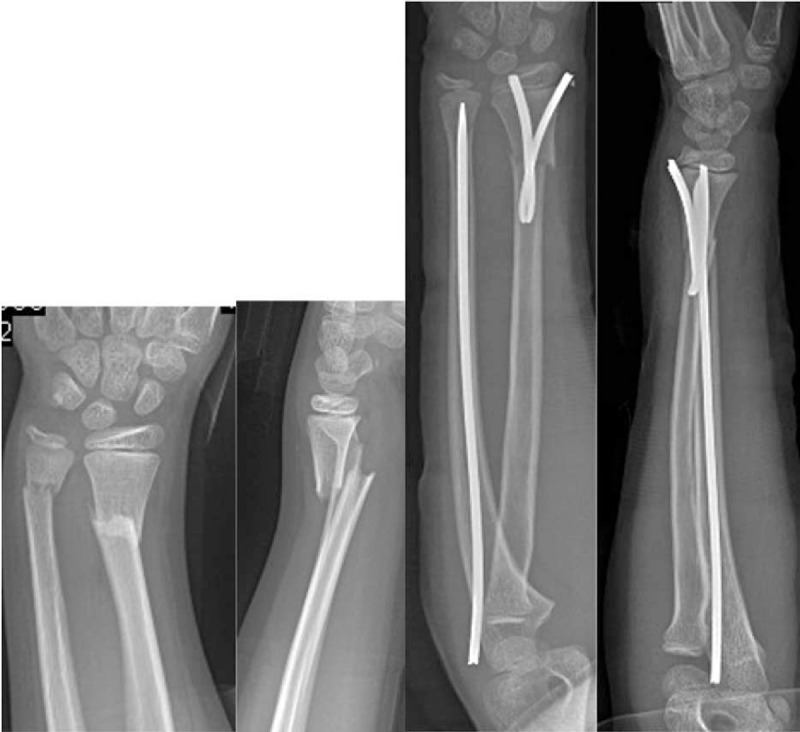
Pre- and postoperative x-rays of displaced distal forearm fracture.
